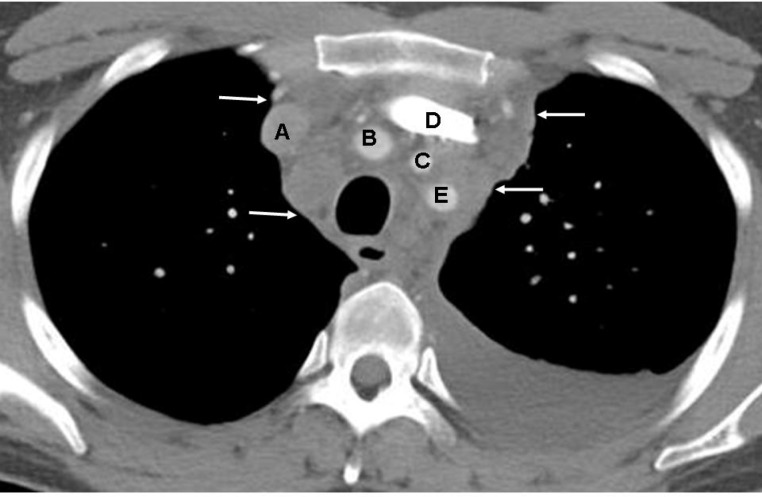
Contrast enhanced CT of the chest of a 25 years old male with Hodgkin lymphoma shows a large confluent mass (arrows) in the anterior mediastinum encasing, but not compressing, the right and left brachiocephalic veins (A and D respectively), brachiocephalic artery (B), left common carotid artery (C) and left subclavian artery (E). Prominent opacification of the left brachiocephalic vein is due to injection of the radiographic contrast in the left arm. Left sided pleural effusion is also seen
